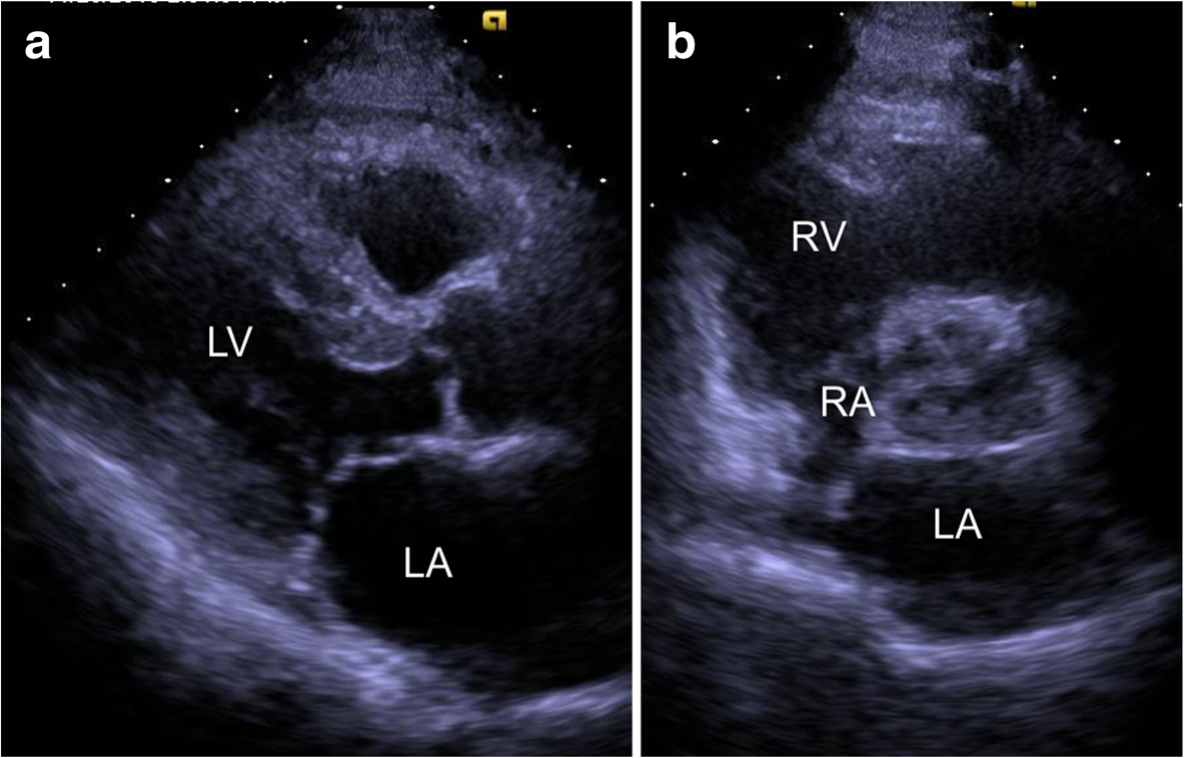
Long-axis (a) and modified short-axis (b) transthoracic echocardiograms before surgical resection, showing no left atrial mass. Abbreviations: *LA* left atrium, *LV* left ventricle, *RA* right atrium, *RV* right ventricle
